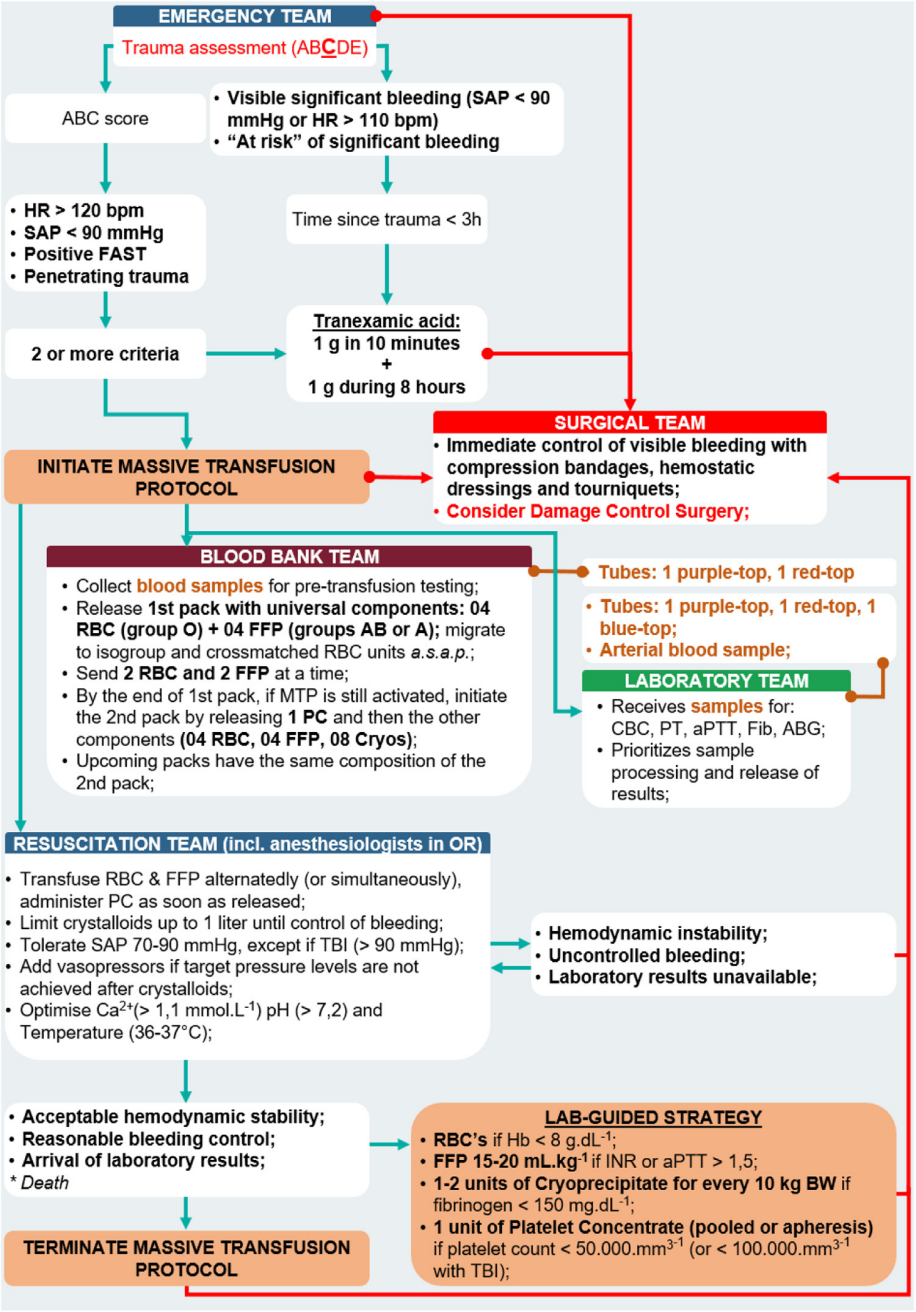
Example of MTP in a Level-1 Trauma Center (Instituto Dr. José Frota, Fortaleza-CE, Brazil). HR, Heart Rate; SAP, Systolic Arterial Pressure; bpm, beats per minute; FAST, Focused Assessment with Sonography for Trauma; RBC, Red Blood Cell Concentrates; FFP, Fresh Frozen Plasma; PC, Platelet Concentrate; MTP, Massive Transfusion Protocol; Cryo, Cryoprecipitate; CBC, Complete Blood Count; PT, Prothrombin Time; aPTT, Activated Partial Thromboplastin Time; TBI, Traumatic Brain Injury; Ca^2+^, Ionized Calcium.
